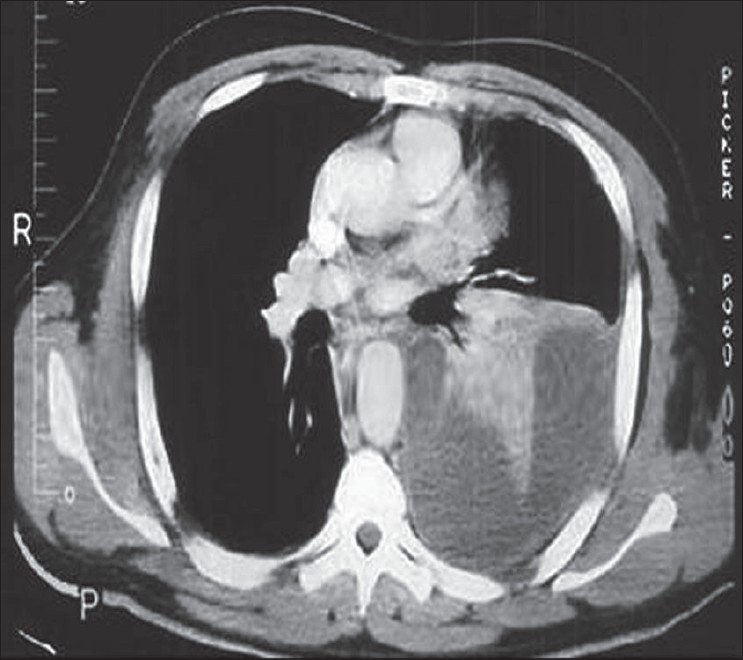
CT scan of the same patient in Figure 1, demonstrating loculation and septation of the pleural space, indicating a phase II empyema
